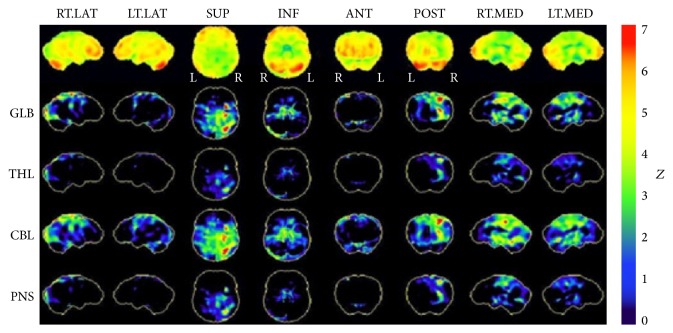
Single-photon-emission computed tomography (SPECT). Cerebral perfusion scan 99mTc HMPAO Z-score reconstructed images. Statistical mapping carried out using the NeuroSTAT programme. Demonstrating asymmetrically decreased activity involving the parietal, occipital, and, to a lesser extent, temporal lobe cortex, greater on the right than on the left. RT.LAT = right lateral; LT.LAT = left lateral; SUP = superior; INF = inferior; ANT = anterior; POST = posterior; RT.MED = right medial; LT.MED = left medial. GLB = global; THL = thalamus; CBL = cerebellum; PNS = pons.
